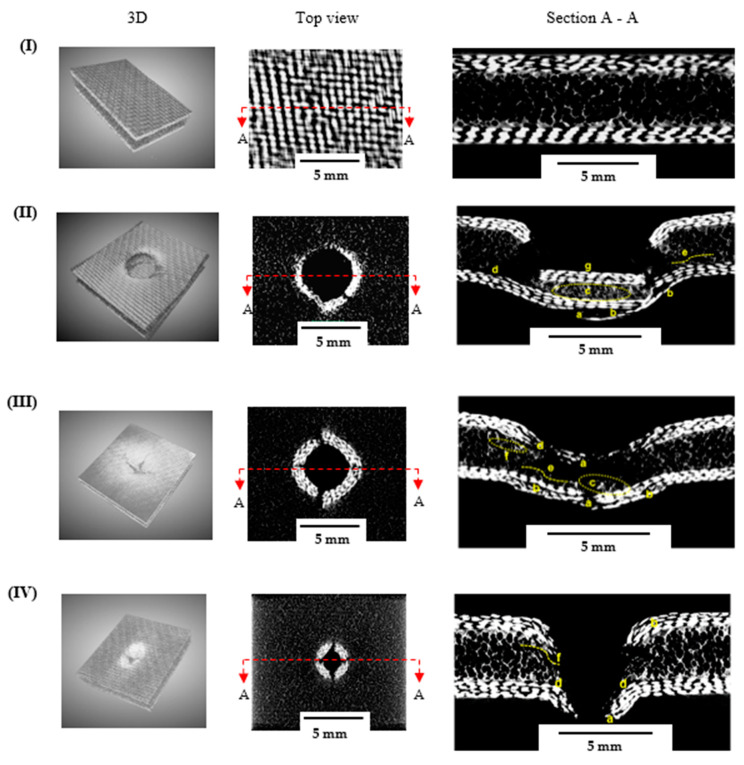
X-ray μ-CT analysis for GS samples: (I) undamaged (II) GSS, (III) GSH, (IV) GSC note; a—fibre breakage, b—delamination, c—core crushing, d—core/face sheet debonding, e—core fracture crack, f—core compression, g—compressed face sheets.
